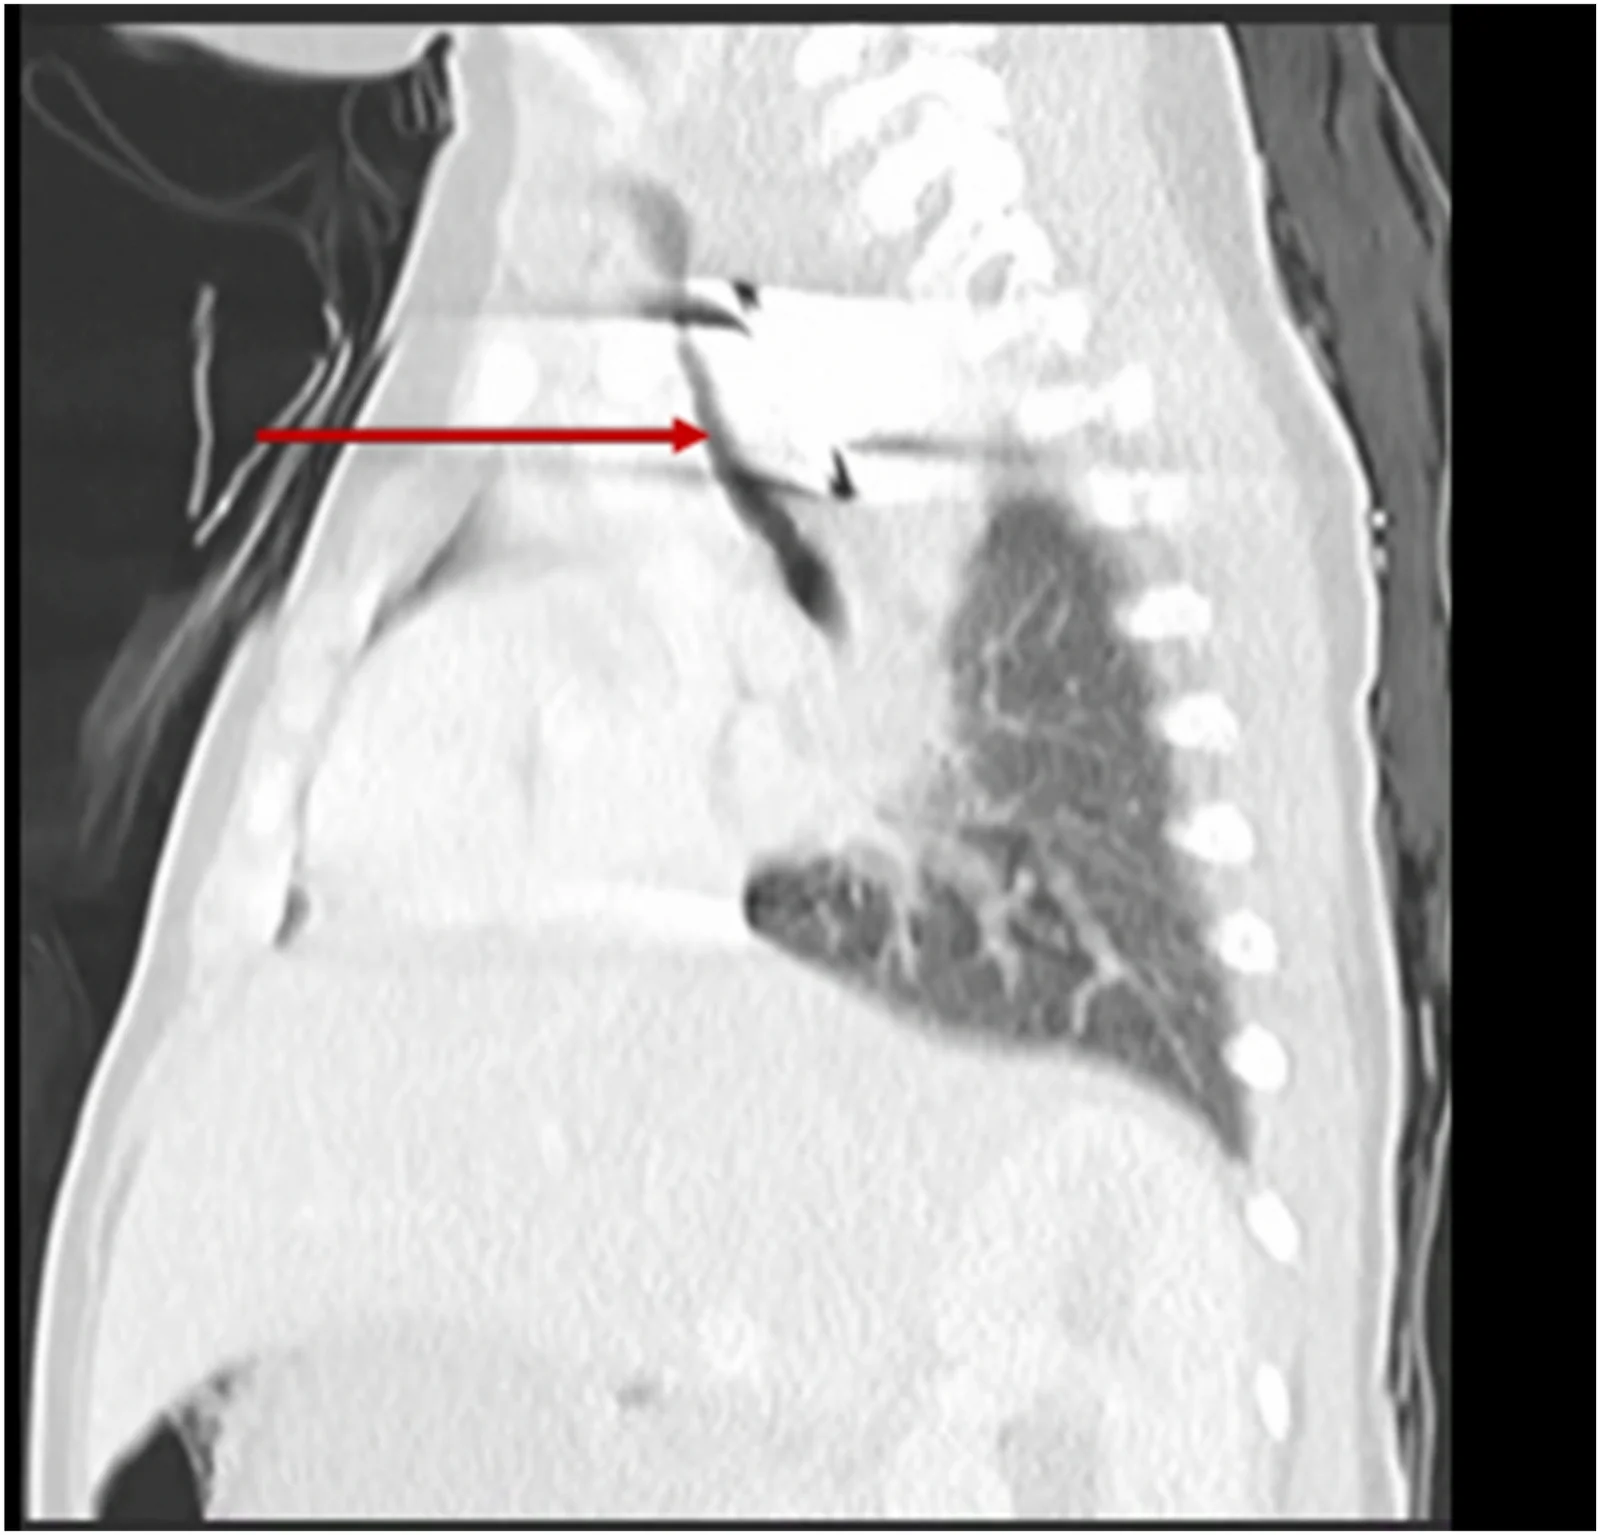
Preoperative CT scan demonstrating a metallic foreign body (indicated by the *red arrow*) within the esophageal wall.
